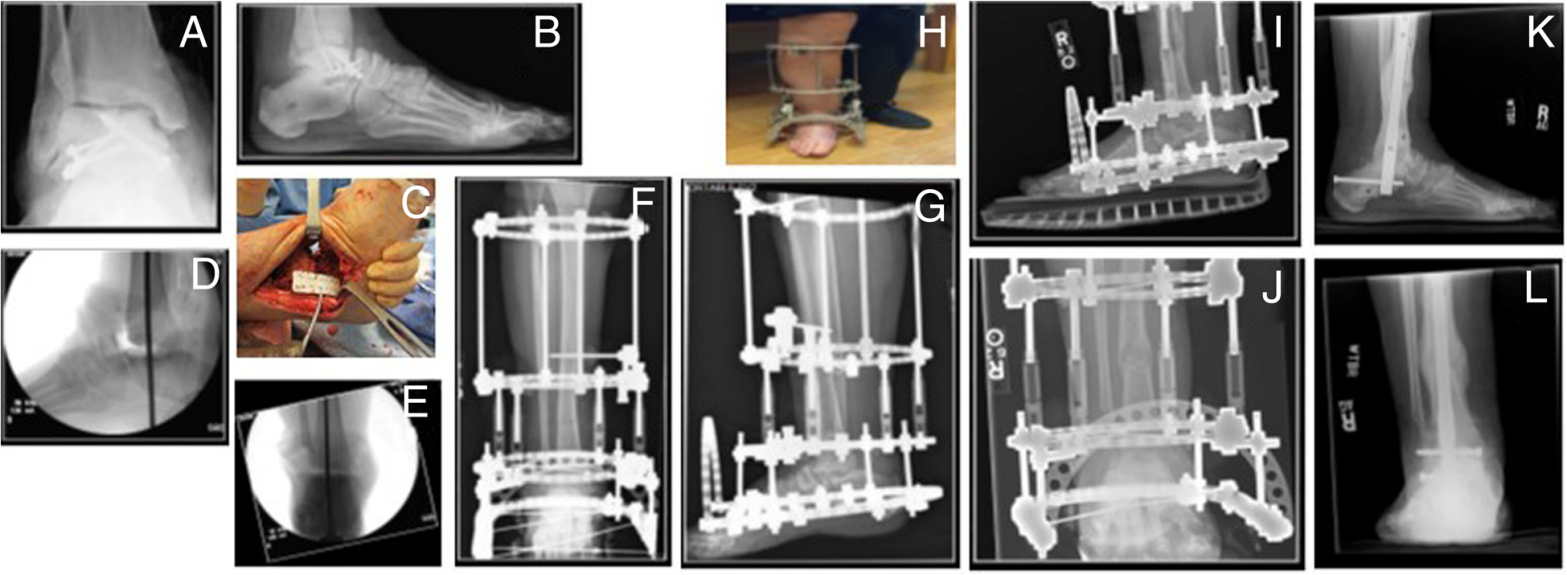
Case #10. Seventy-four-year-old F 9 months s/p motor vehicle accident with closed talus fracture. a, b Preoperative radiographs demonstrating talar collapse and AVN. c Intraoperative image post talectomy. d, e Intraoperative fluoroscopy of tibiocalcaneal fusion site alignment. f, g AP and lateral radiographs demonstrating frame construct for tibiocalcaneal fusion and DTC. h–j Clinical and radiographic appearance of frame construct demonstrating regenerate formation. k, l Final postoperative AP and lateral radiographs following distal tibial lengthening, tibiocalcaneal fusion, and intramedullary nailing
